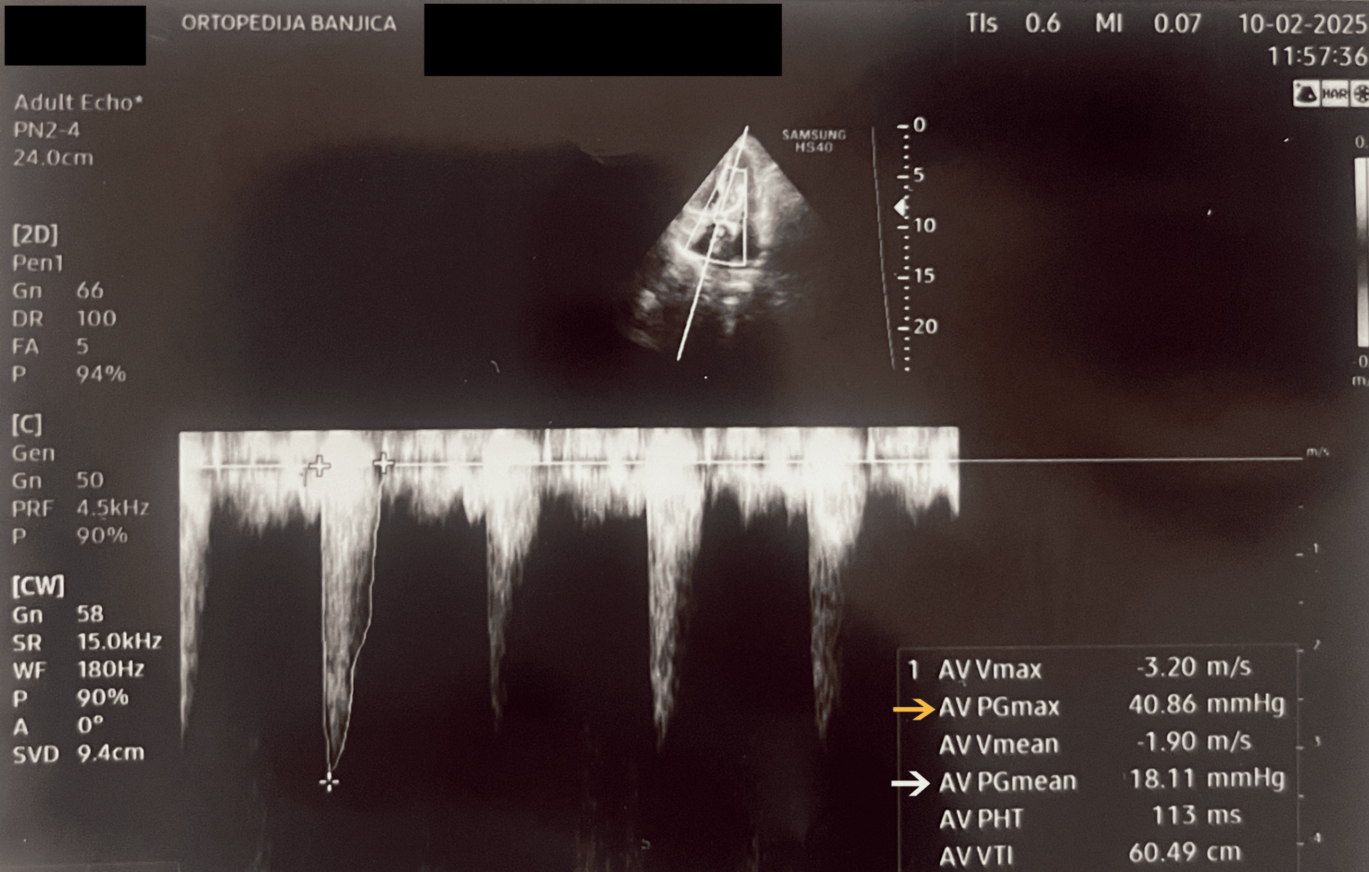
Echocardiographic image of the pressure gradient through the aortic valve In the image, the yellow arrow indicates the maximum pressure gradient through the aortic valve (40.86 mmHg), while the white arrow represents the mean pressure gradient (18.11 mmHg).
